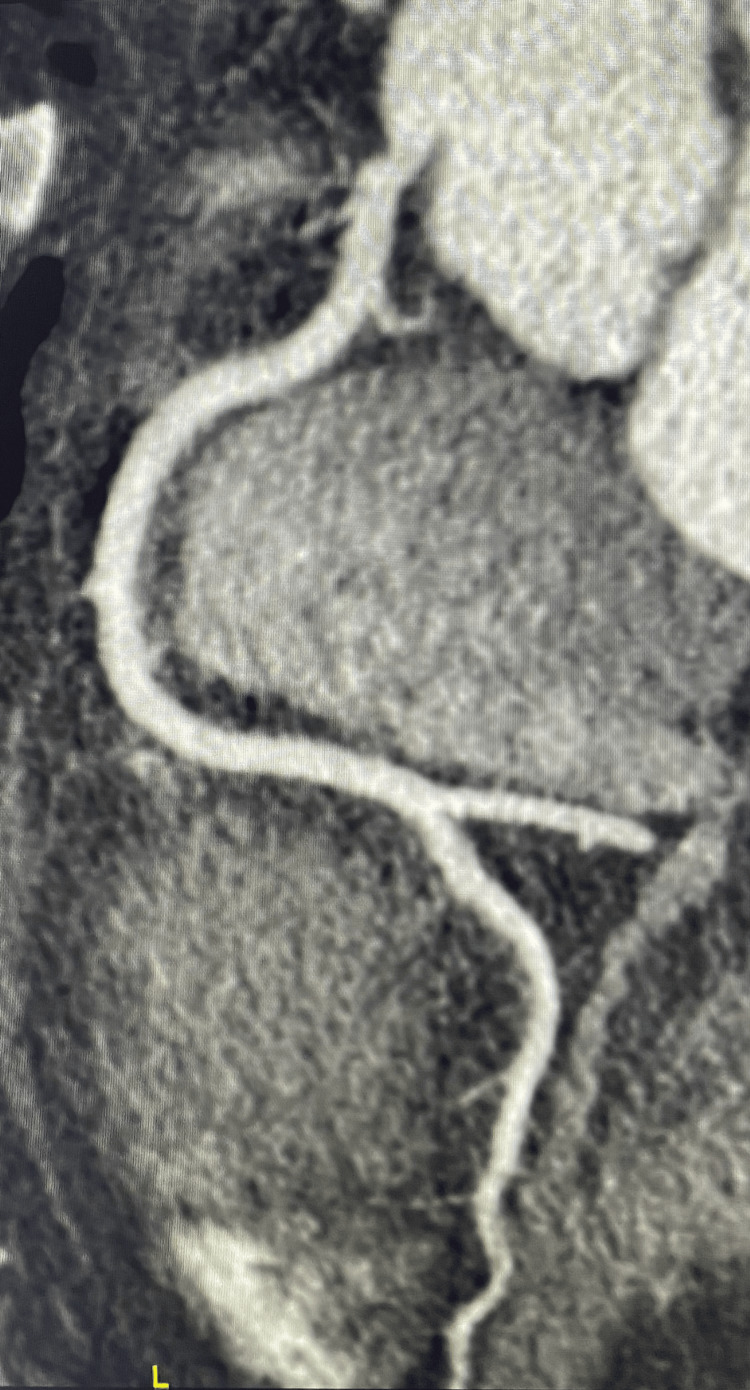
CT coronary angiogram showing normal right coronary artery CT: computed tomography
